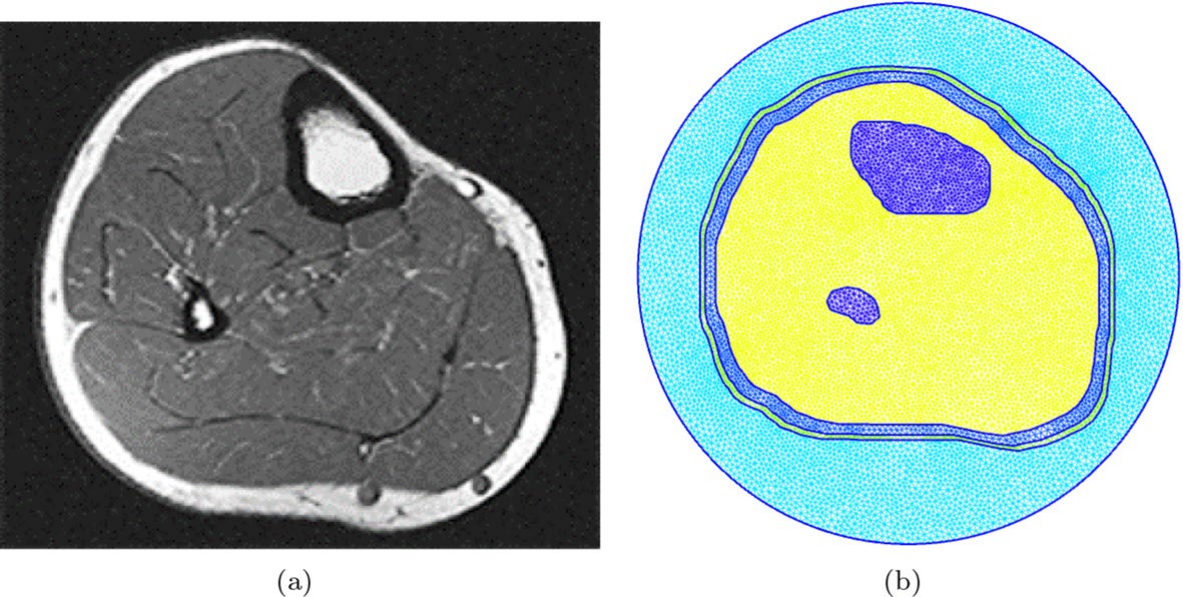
The cross-sectional MRI image and the corresponding finite-element model and mesh: a MRI image [50], and b finite-element model
